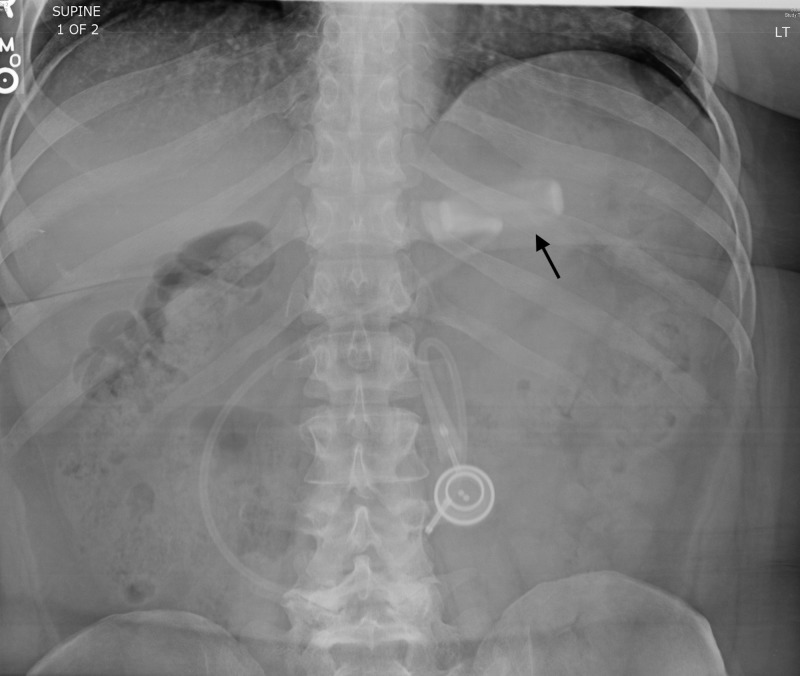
Abdominal radiograph demonstrating a horizontal positioning of the lap band (arrow).
